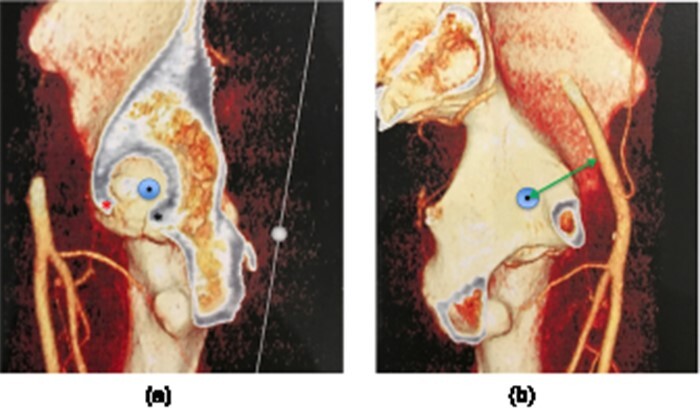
Three-dimensional reconstruction of a CT angiography demonstrating the LT acetabular attachment (large circle). (A) View of the cotyloid fossa and (B) view of the quadrilateral plate of the pelvis demonstrating the clearance distance (arrow) between the center of the acetabular LT tunnel (small circle) and obturator vein.
